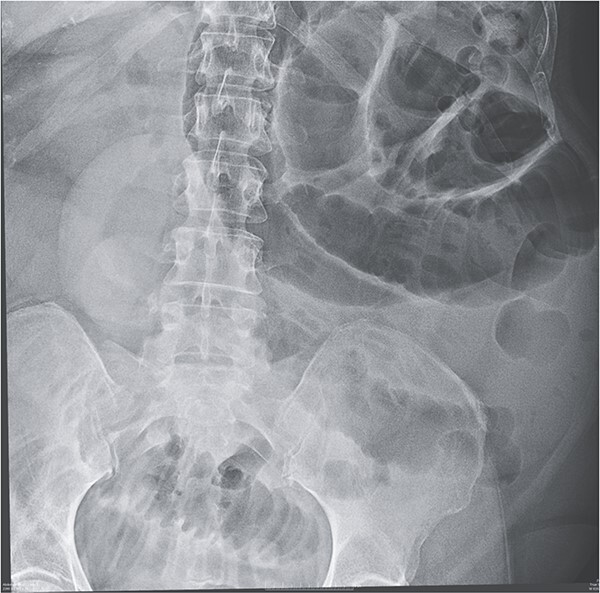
X-ray showing multiple dilated small bowel loops.
